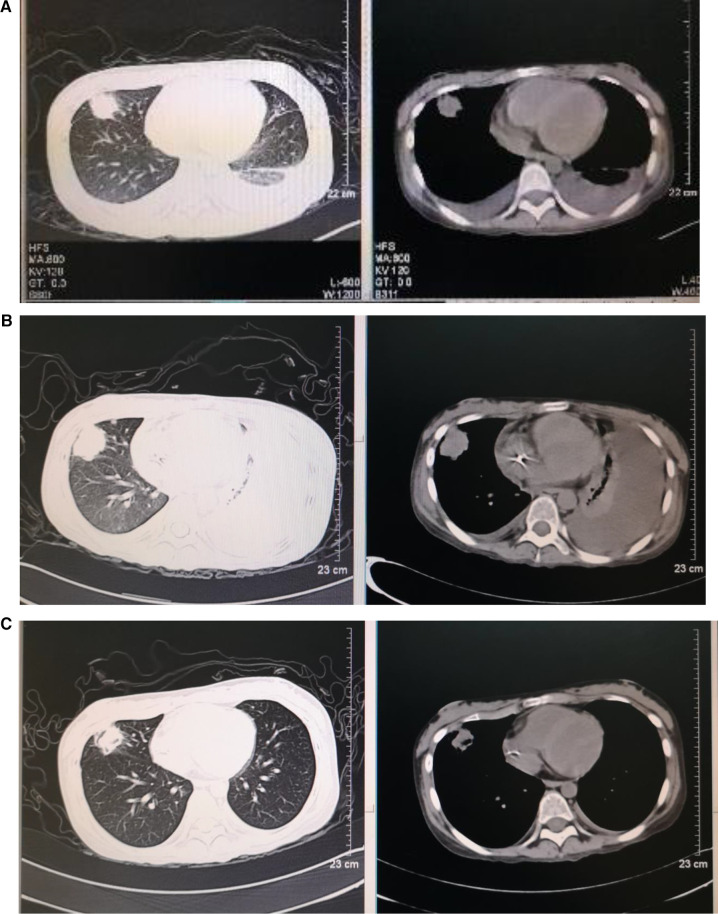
Dynamic images of pneumonia before and after transplantation (A–C). Chest high-resolution computed tomography: (A) 3.3 × 2.7 cm nodular shadow in the right upper lung, with bilateral pleural effusion on day −7 before HSCT, (B) 3.3 × 3.1 cm nodular shadow in the right upper lung, with bilateral pleural effusion and left atelectasis on day +22 after HSCT, and (C) 2.3 × 2.0 cm nodular shadow in the right upper lung on day +59 after HSCT.
